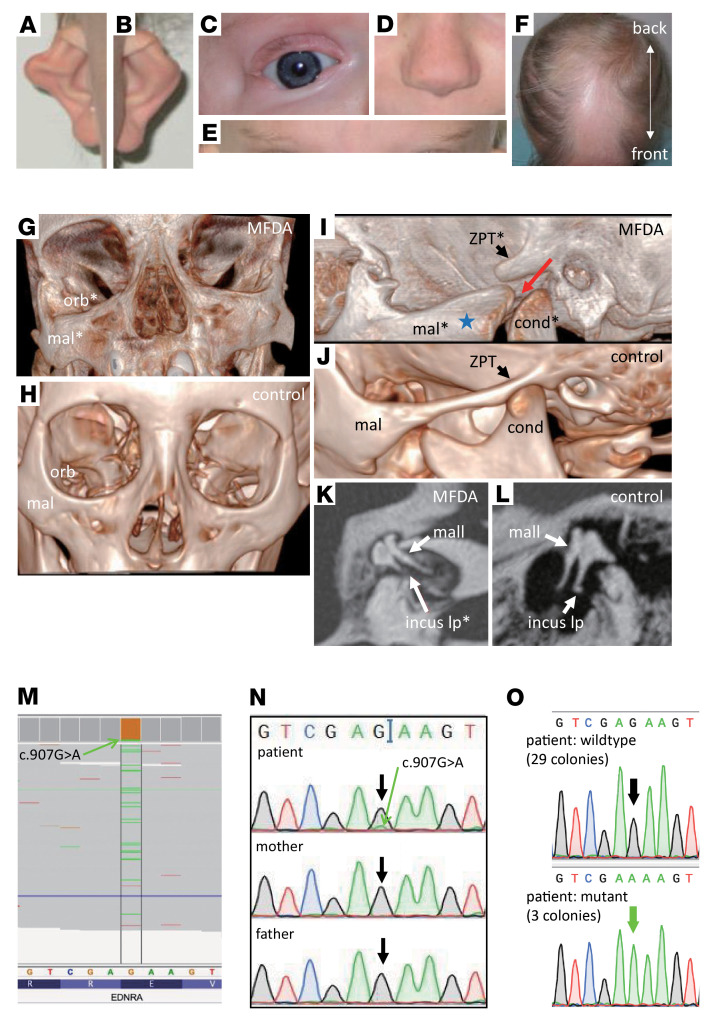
A patient with MFDA harboring the *EDNRA* mutation p.E303K, that occurred postzygotically. (A–F) Dysmorphic facial features and alopecia of the patient (see text for details). (G–J) High resolution craniofacial 3D CT-scans of the patient with MFDA (G and I) and an age-matched control (H and J). (G and H) Frontal views show hypertrophic, dysplastic, and asymmetric malar bones and an abnormal orbital floor in the patient (G) compared with the control (H). (I and J) Volume-rendering sagittal reconstructions of the temporomandibular articulations show a novel, tripartite temporo-mandibulo-malar articulation on the left side (red arrow) with increased malar bone volume (blue star) in the patient (I) compared with the control (J). (K and L) Oblique axial reconstructions of CT scans of the incus and malleus showing hypoplasia of the long process of the incus in the patient (K) compared with a control (L). (M) Next-generation sequencing reads showing mosaicism for the *EDNRA* variant c.907G>A (8%–11% of reads, in green) in the patient’s peripheral blood DNA. (N) Sanger sequencing of *EDNRA* amplified by PCR from leukocyte DNA of the patient and her parents, demonstrating low -evel mosaicism for the variant c.907G>A (green arrow) in the patient. (O) Sequencing of PCR products amplified from individual bacterial colonies, following transformation with cloned *EDNRA* PCR fragments from the patient, indicating 3/32 colonies (9 %) harboring the variant (green arrow) while 29 colonies harbored the WT allele (black arrow). Abbreviations: orb, orbital floor; mal, malar bone; cond, condyle of the mandible; ZPT, zygomatic process of the temporal bone; mall, malleus; incus lp, long process of the incus. An asterisk indicates structures that are dysmorphic compared with normal.
